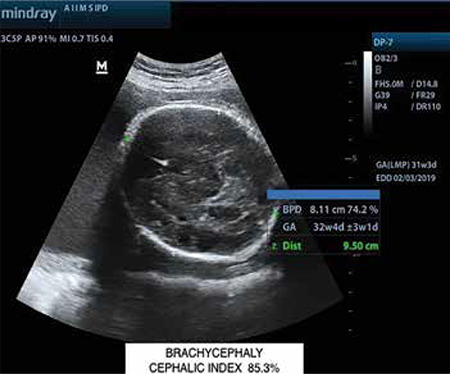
Ultrasound image (2-dimensional) of fetal head showing brachycephaly with cephalic index more than 85% [Biparietal diameter (BPD): 8.11 cm, Occipito-frontal diameter (OFD): 9.5 cm, Cephalic index (BPD/OFD) x100: 85.4%] BPD: Biparietal diameter
